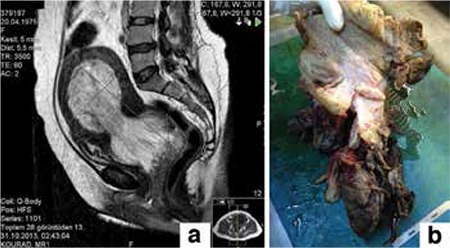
a: Magnetic resonance images showed a bilobular solid heterogeneous mass that filled the endometrial cavity. b: Macroscopically, the tumor was polipoid lesion extending into the endometrial cavity
